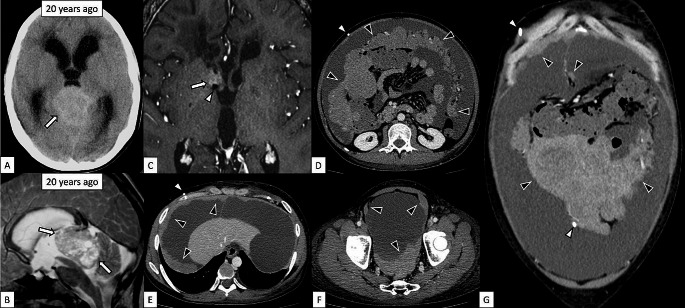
Intracranial pure germinoma and diffuse peritoneal dissemination. A, B: Nonenhanced CT and T2-weighted sagittal image of the primary pineal pure germinoma 20 years ago. There is a high-attenuated and hyperintense mass with multiple cystic components in the pineal region (A, B, arrows). C: Fat-suppressed 3-dimentional contrast-enhanced T1-weighted imaging of the brain shows a recurrent tumor with contrast enhancement in the right frontal horn (arrow) adjacent to the tip of the VPS (white arrowhead). D–G: Contrast-enhanced abdominal CT images (D–F: axial imaging, G: coronal imaging) show the VPS (white arrowheads) and diffuse confluent multinodular masses with homogeneous enhancement with approximately 70 Hounsfield Unit, except for the suspected central necrosis in the largest omental mass, along the abdominal wall and greater omentum (black arrowheads). Massive ascites with approximately 20 Hounsfield Unit are also demonstrated
